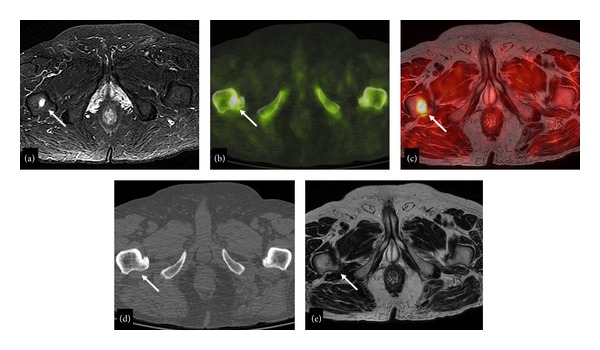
78-year-old man with a bone metastasis in the right proximal femur. The bone lesion (arrow) is well detectable on STIR (a), ^18^F-Fluoride-PET/CT (b), and fused ^18^F-Choline-PET/MRI axial images, while it is not visible on T2-weighted (d) and CeCT (e) axial images.
